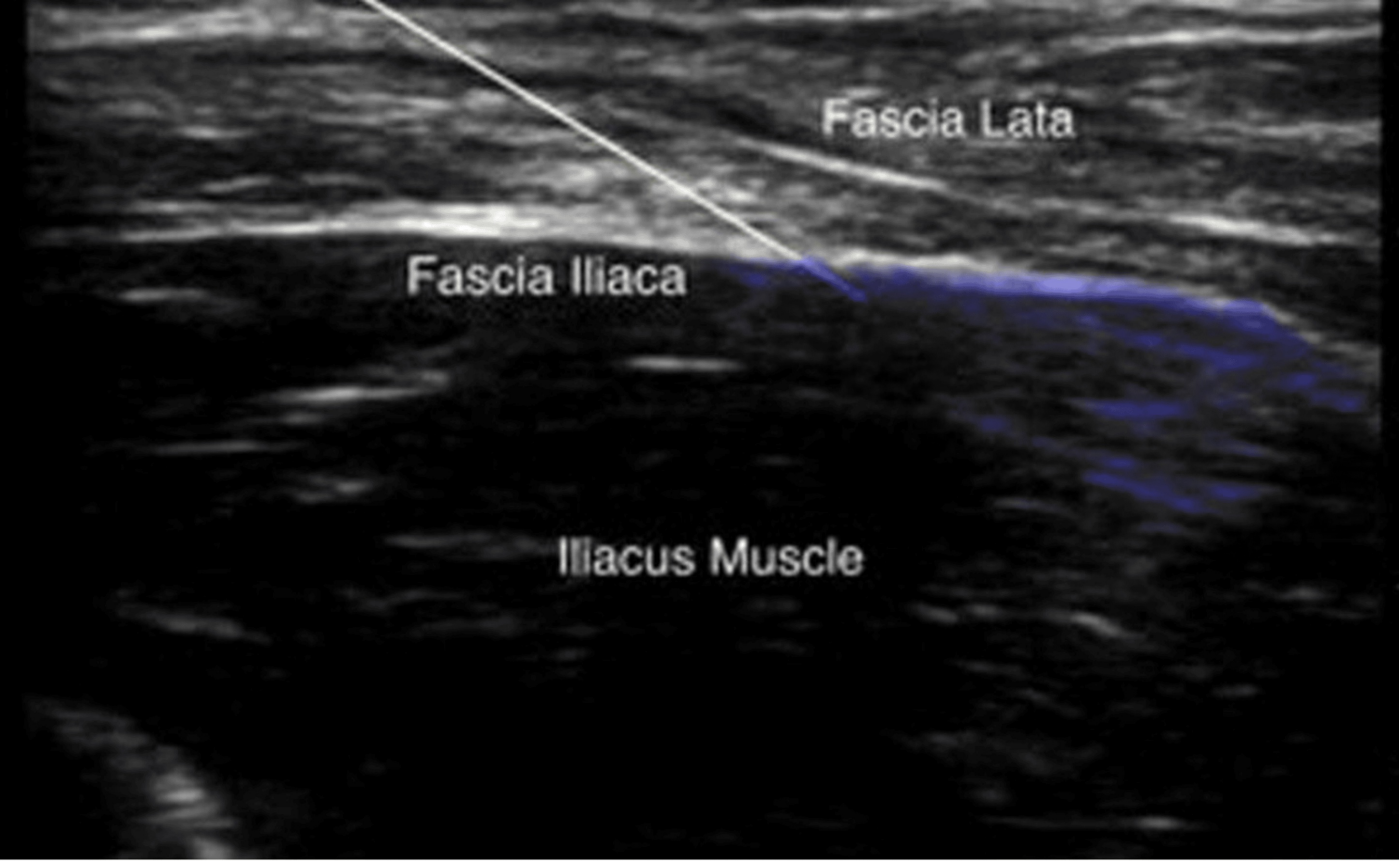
Ultrasound image of FICB block
